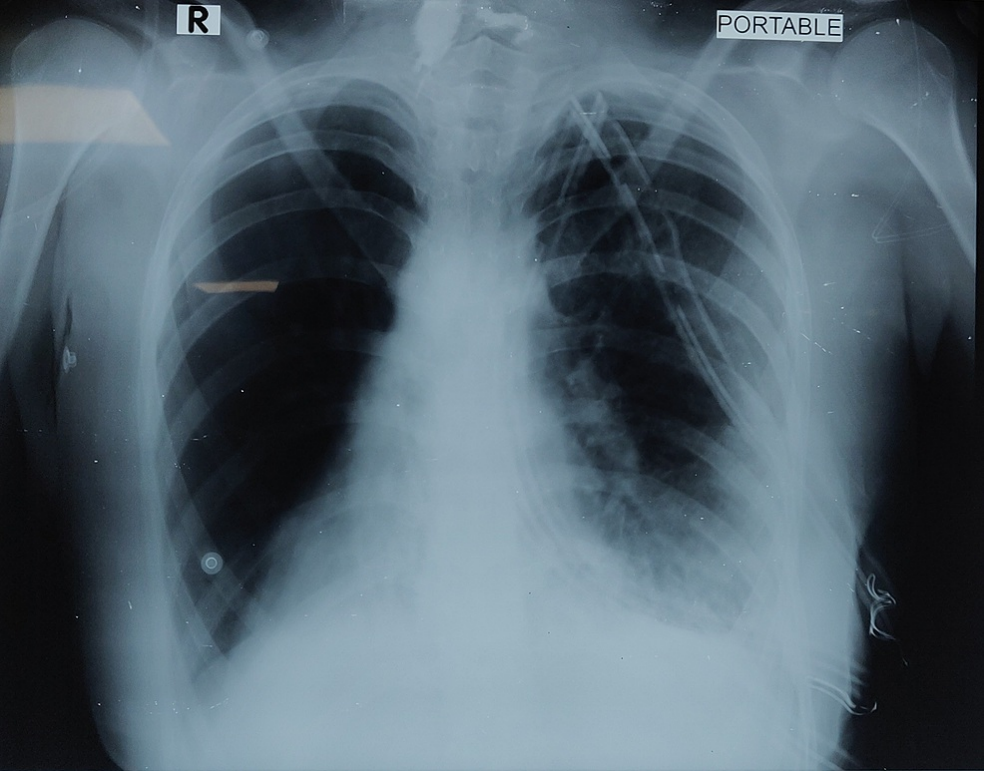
Postoperative chest X-ray.
